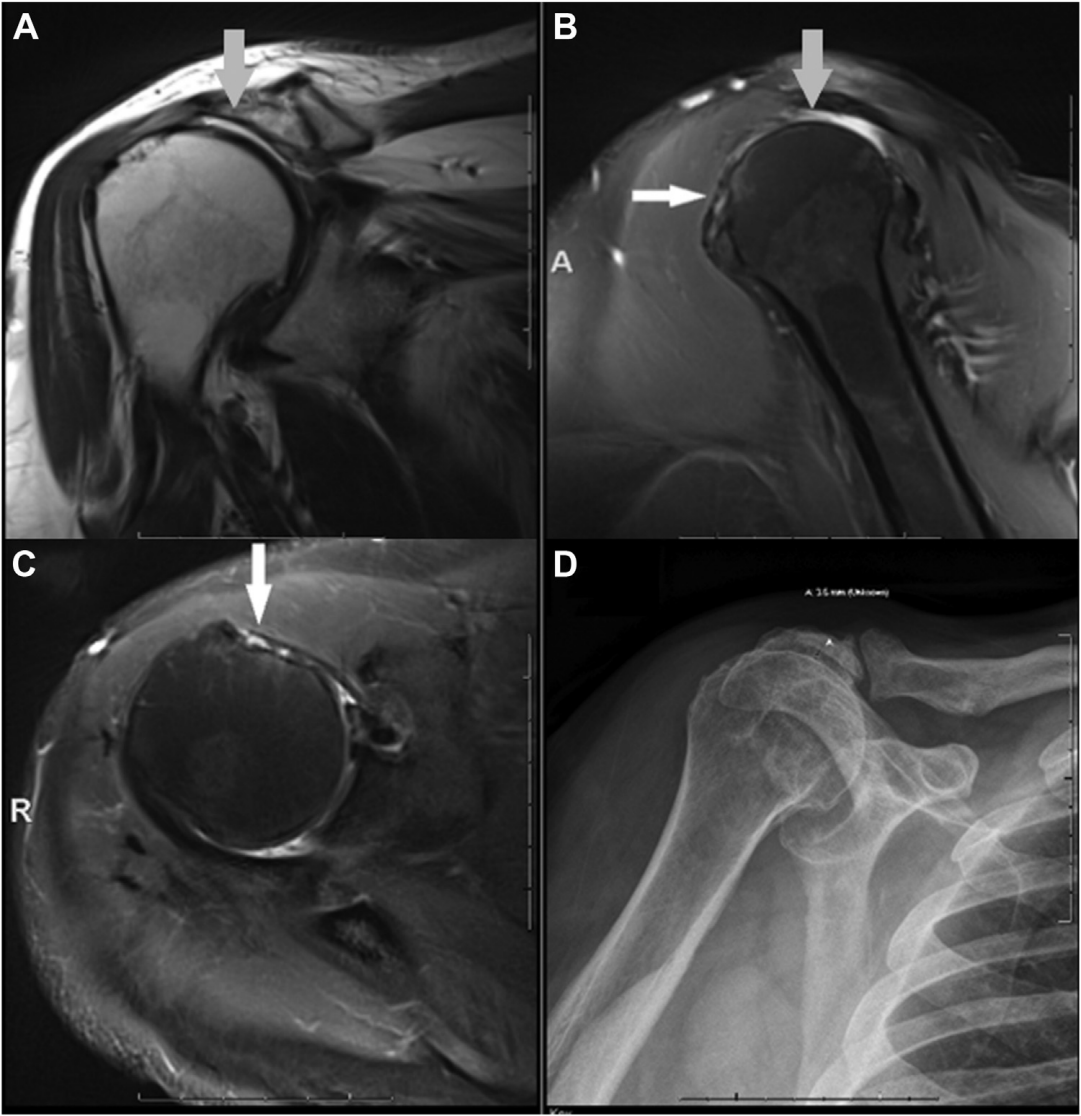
A 3T MRI scan of the right shoulder on a 48-year-old female patient using routine protocol. (A) Coronal T2-weighted image and (B) sagittal proton density fat saturated image demonstrating complete full thickness tears of the supraspinatus tendon (*gray arrows*). (B) Sagittal proton density fat saturated image and (C) axial proton density fat saturated image demonstrating absent PLHB tendon in the bicipital groove in keeping with full thickness tear (*white arrows*). (D) Corresponding frontal radiograph shows superior humeral head migration and narrowing of the acromiohumeral distance (3.5 mm). *MRI*, magnetic resonance imaging.
